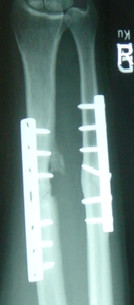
X-ray of the right forearm 12 months after ORIF of a 57 year old man showing a persistent non-union of the radius.
